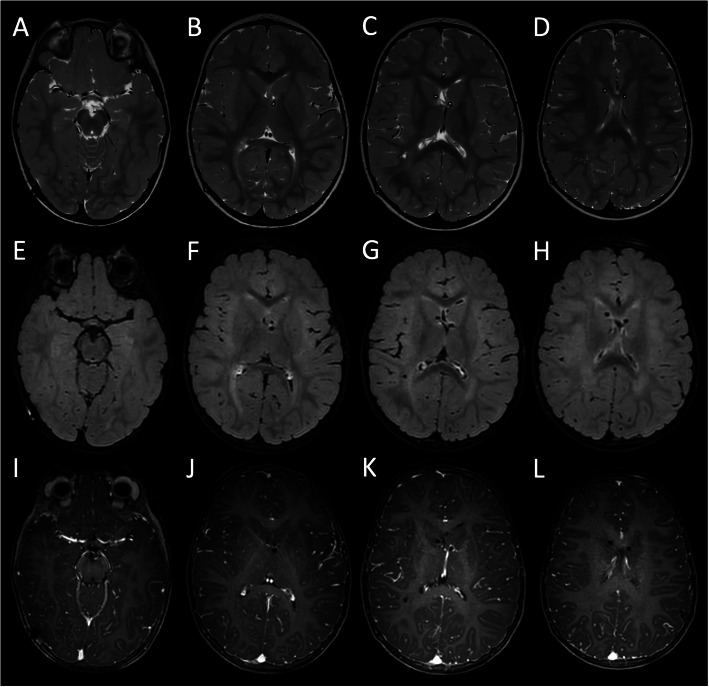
Brain MRI performed 6 months after clinical onset. Axial T2-weighted (a-d), contrast-enhanced FLAIR (e-h) and T1-weighted (i-j) images demonstrate complete resolution of the periventricular white matter signal alterations, leptomeningeal enhancement and reactive dural enhancement. Note the absence of brain lesions
